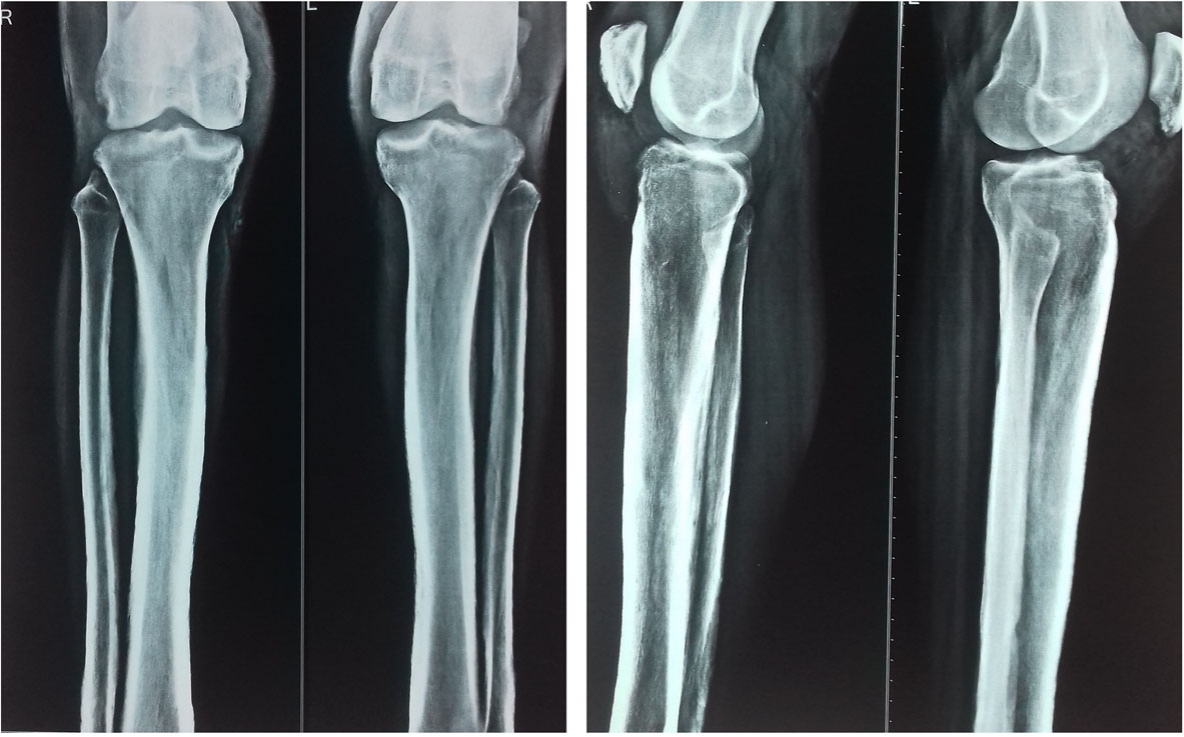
Radiograph of bilateral knees showing periosteal hypertrophy with subperiosteal bone formation
